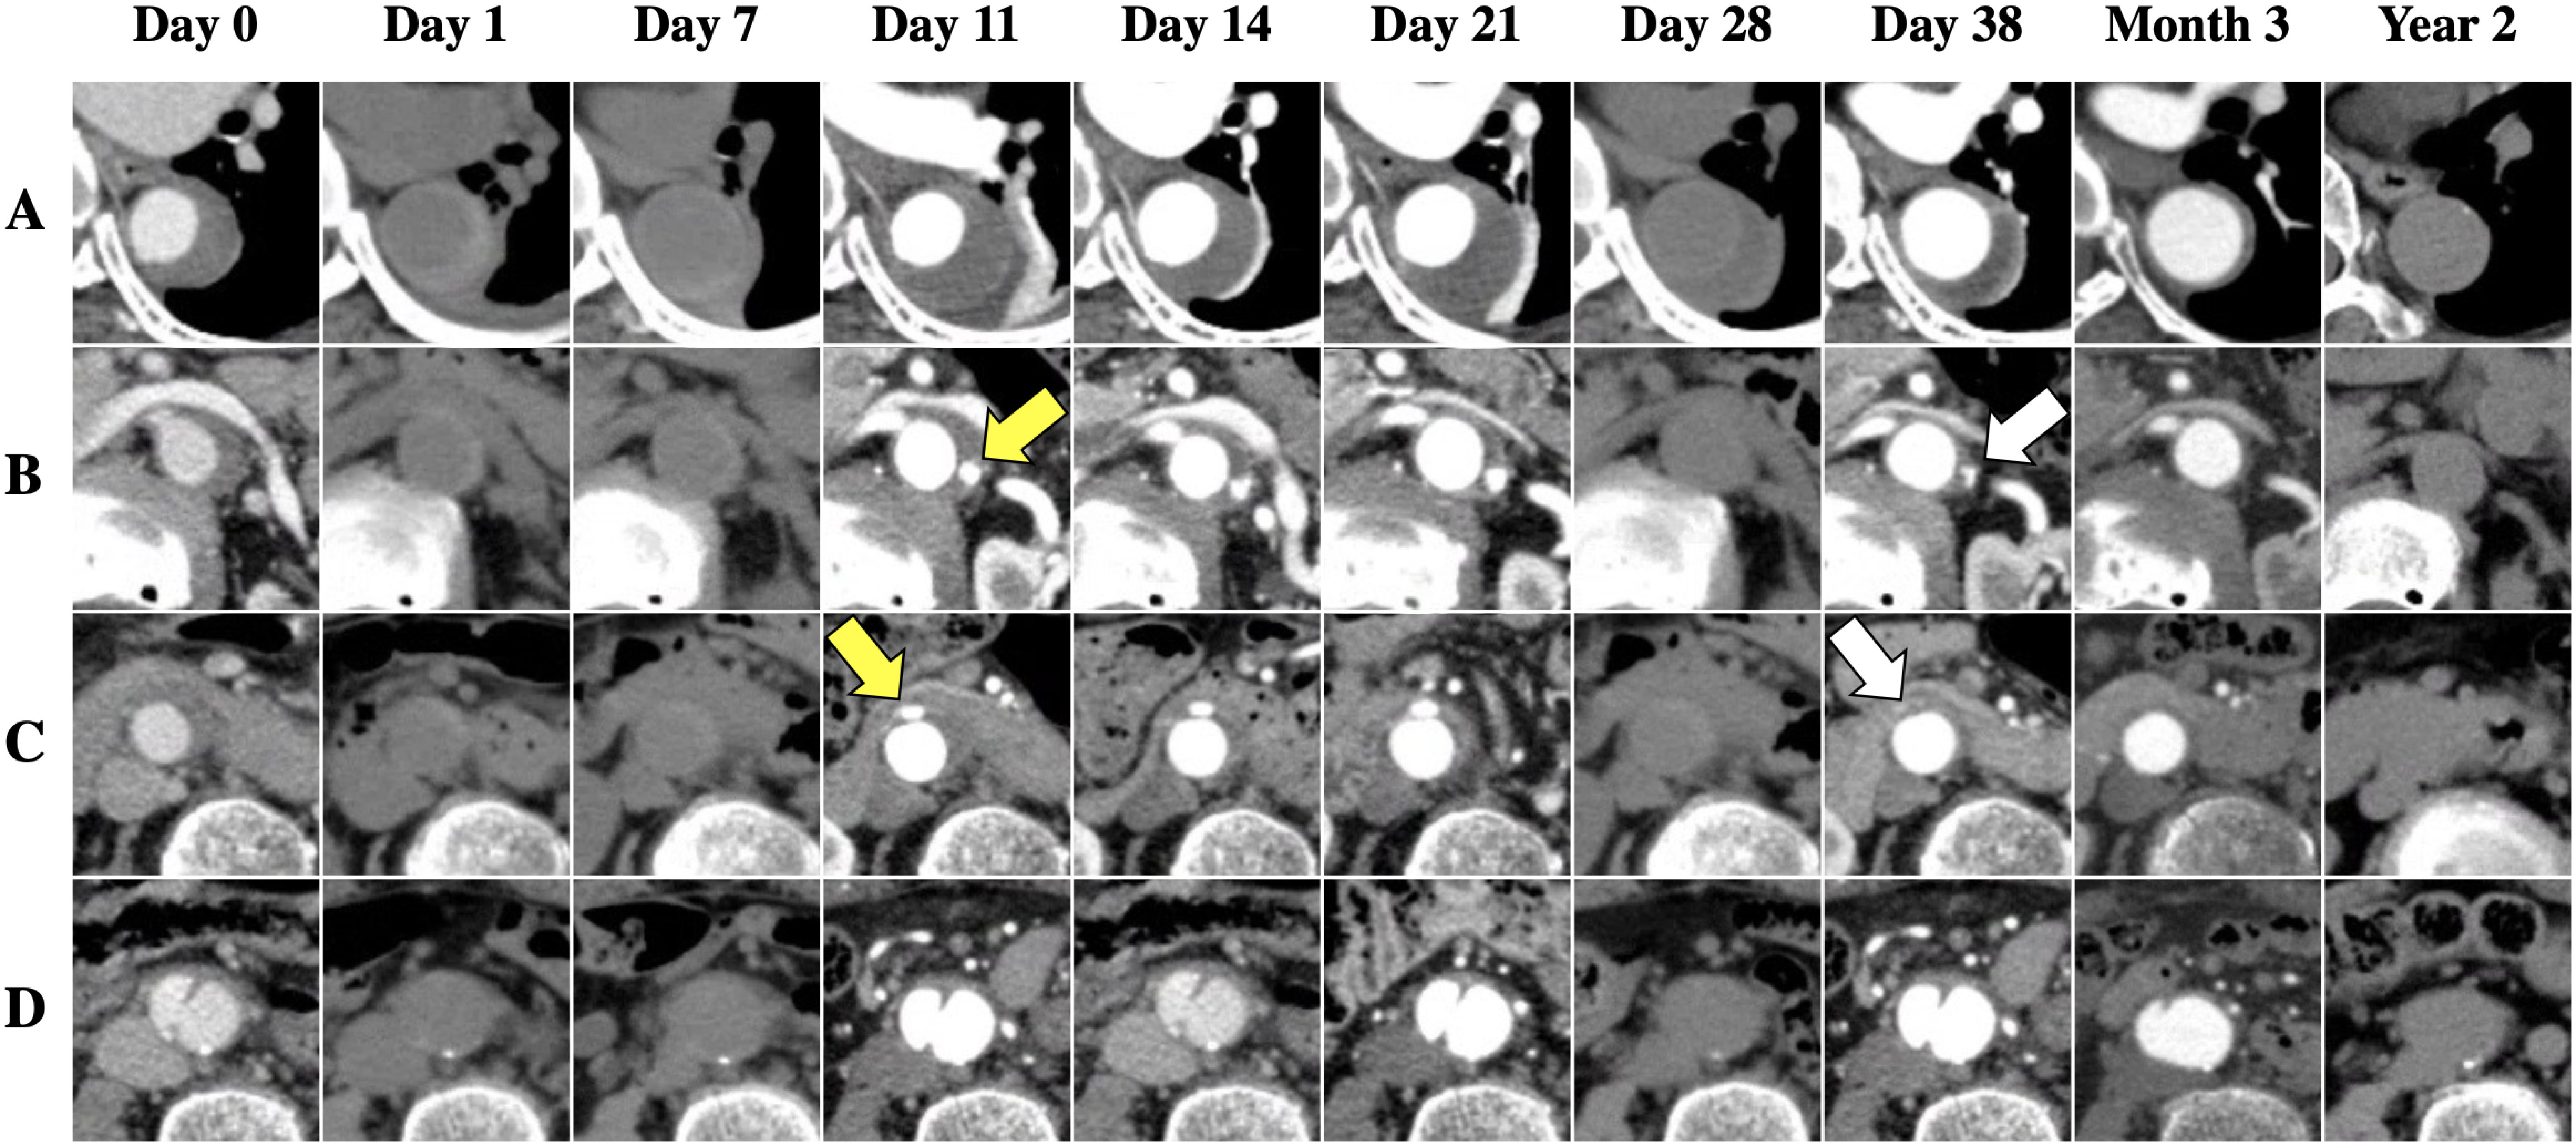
Fig. 1 Serial computed tomography (CT) scans (A: descending thoracic aorta level; B: left middle suprarenal artery level; C: right gonadal artery level; D: abdominal intimal defect level) show regression and re-expansion of the thrombosed false lumen and newly emerged collateral retrograde flow (yellow arrows) from the aortic branches on day 11. On day 38, enhanced CT demonstrate decreased flow from the left middle suprarenal artery, and that from the right gonadal artery disappeared (white arrows).
